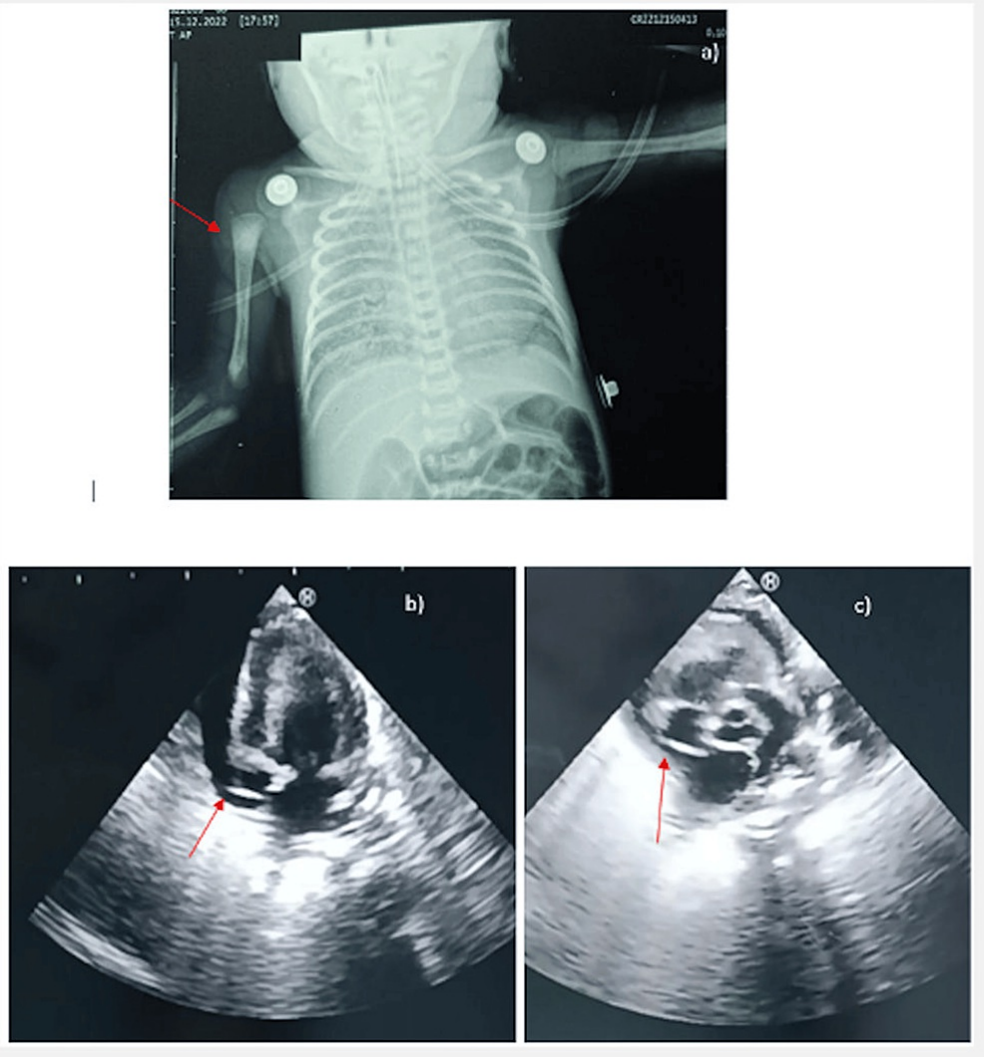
Echocardiogram and chest radiograph of Case 3 a) Chest X-ray performed in a supine position to confirm the PICC position but unable to reveal catheter tip position; b) PCE with the PICC tip within the right atrium; c) PICC tip within located the right atrium PICC: Peripherally inserted central catheter; PCE: Pericardial effusion
